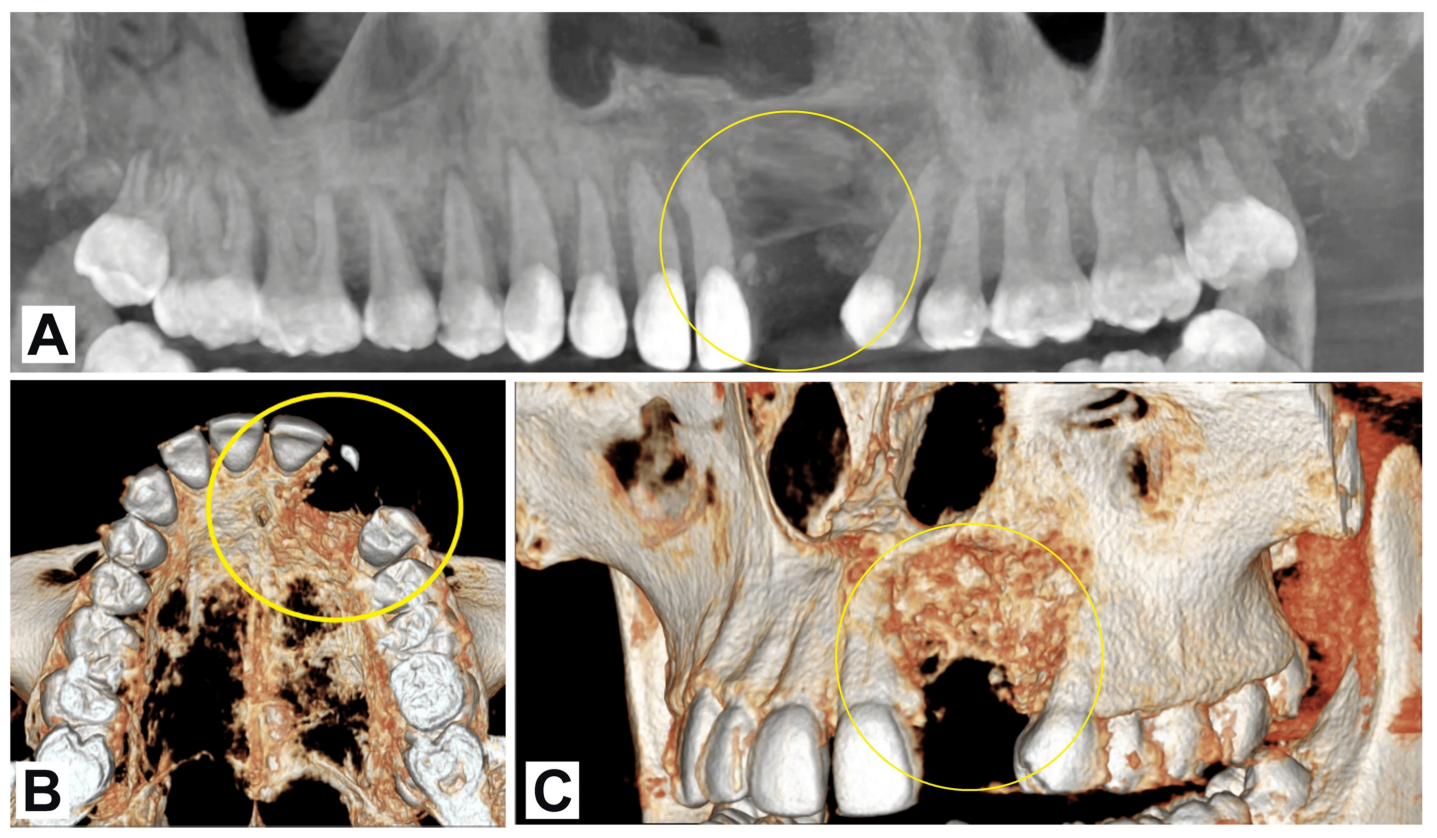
Postoperative follow-up CBCT: A) Panoramic view, B) 3D reconstruction-occlusal view, and C) reconstruction-frontal view CBCT: Cone-beam computed tomography
